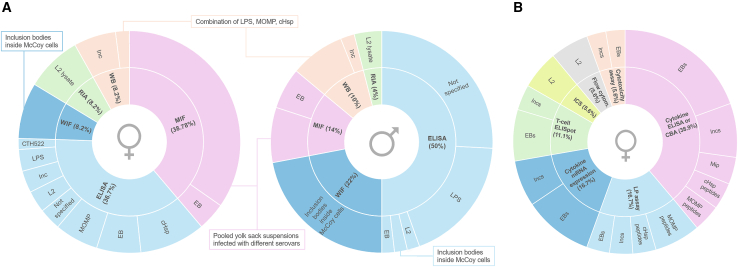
Immunoassays for detecting immune biomarkers in urogenital secretions Overview of different immunoassays for detecting immune biomarkers in urogenital secretions. Percentages indicate proportion of articles using the specified assay. Per immunoassay, different antigens that were used are given in the outer circle (proportional to the number of assays). CBA, cytokine bead array; Incs, inclusion proteins; LP assay, lymphoproliferation assay; L2, CT serovar L2 (lymphogranuloma venereum); Mip, macrophage infectivity potentiator; MOMP, major outer membrane protein; WB, western blot assay. (A) Overview of immunoassays used for detection of antibodies in female (left) and male (right) genital secretions. Studies including both female and male participants were included in both proportions. (B) Overview of different immunoassays used for measuring antigen-specific cellular immune responses in female urogenital secretions.
